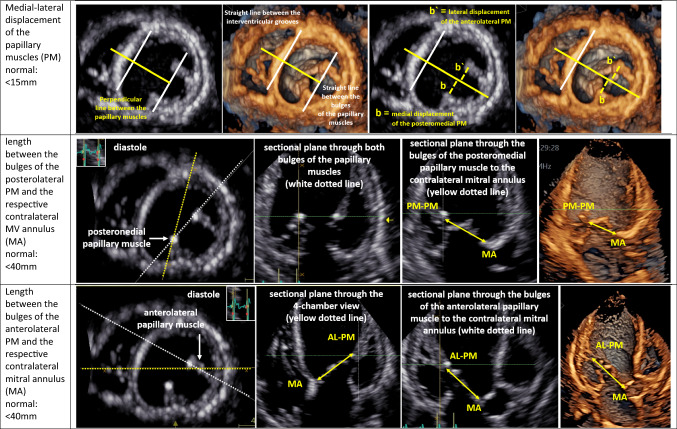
Echocardiographic parameters characterizing left-ventricular (LV) remodelling in MR patients using conventional 2D echocardiography or 3D TTE
